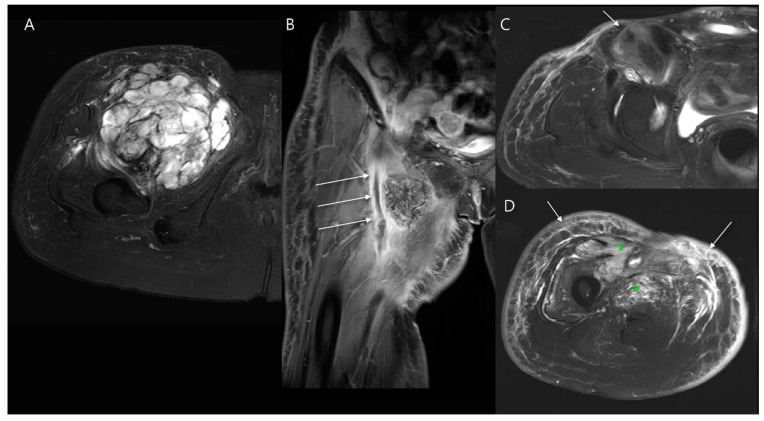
Wound infection. (A) Preoperative axial T2-weighted fat-suppressed MR image shows a myxofibrosarcoma in the proximal thigh. Neoadjuvant chemotherapy was administered, followed by wide excision, femoral nerve neurolysis, and a femoral-femoral bypass procedure. (B) Postoperative coronal T1-weighted fat-suppressed enhanced MR image shows an internal thrombus within the bypass (arrows). (C,D) Axial T2-weighted fat-suppressed images demonstrate herniation of the small bowel through the large defect (arrow in (C)), along with cellulitis (arrows in (D)) and myositis (asterisks in (D)).
